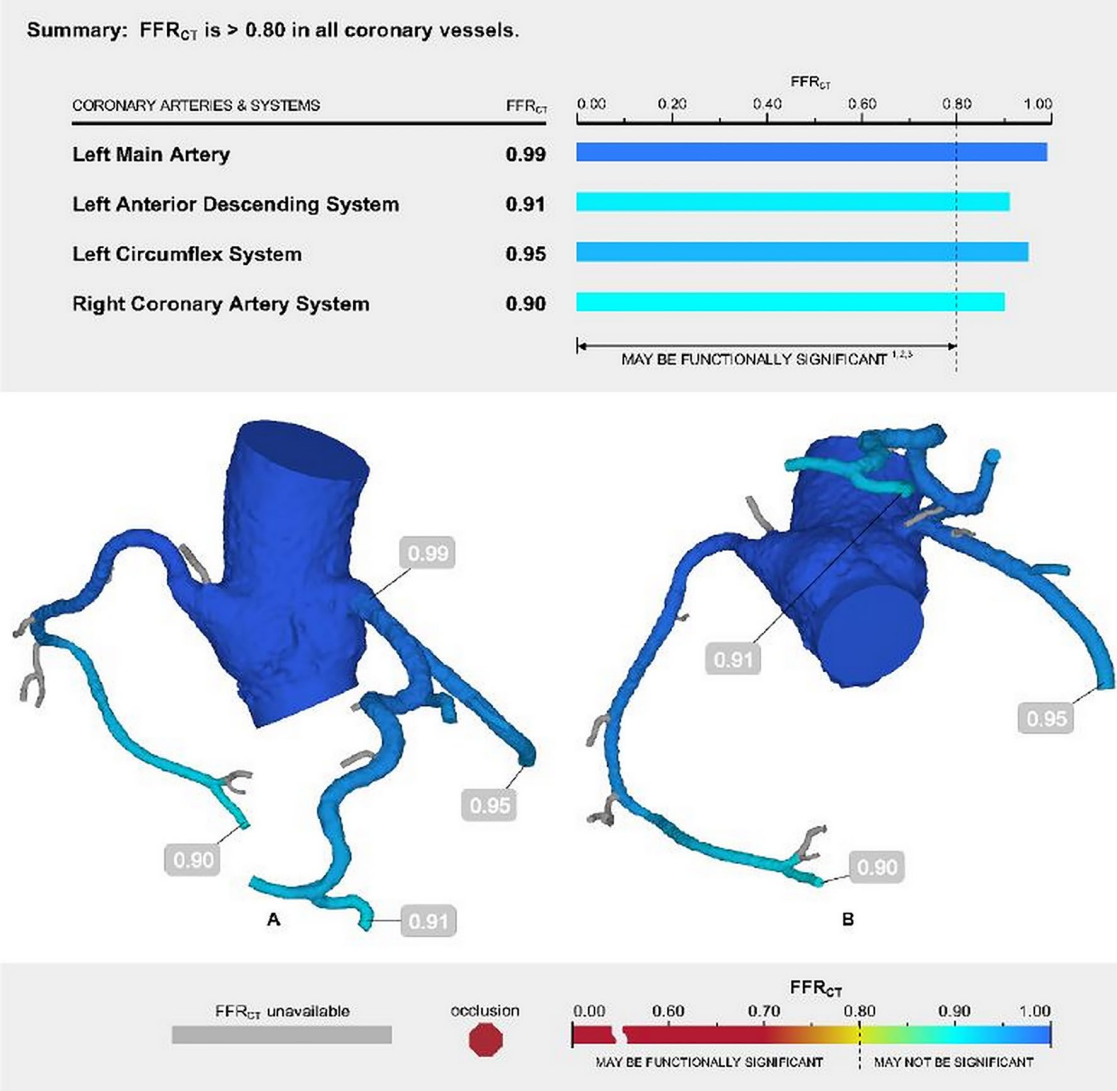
Normal CT-FFR report for a 59-year-old man with atypical chest pain. The CT-FFR report shows the coronary arteries color-coded according to their CT-FFR values. In this patient, all the major coronary arteries show normal values (> 0.8). *CT* computed tomography, *FFR* fractional flow reserve
